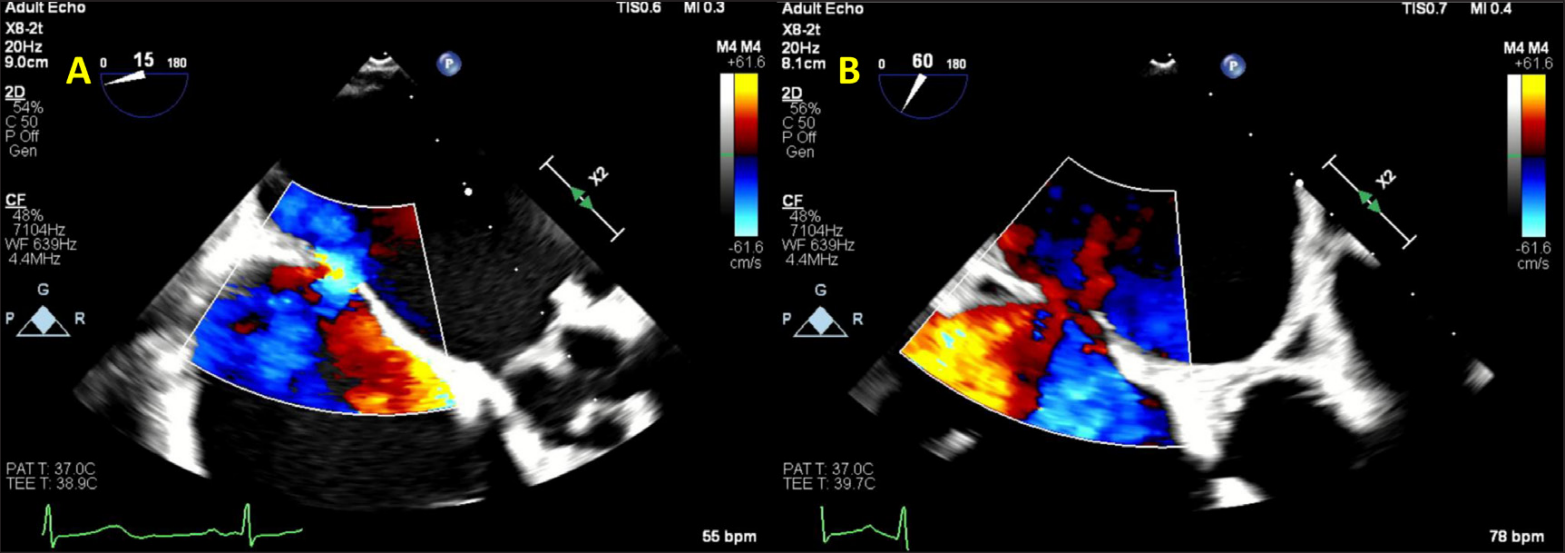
Transesophageal echocardiogram showing (A) residual iatrogenic atrial septal defect (ASD) with bidirectional shunt from prior transcatheter mitral valve replacement that was complicated by paravalvular leak (PVL). (B) During attempt to close the PVL, the patient developed intractable hypoxia and was found to have worsening ASD with bidirectional shunt due to enlargement of the ASD size. See Figure 6 A videoclip at https://youtu.be/Zfbb80XK-jI and Figure 6 B videoclip at https://youtu.be/em48KZ8hbJY.
